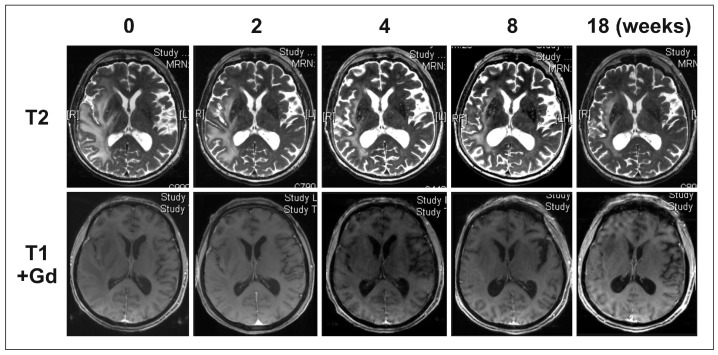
Exemplary magnetic resonance imaging (MRI) of Patient 7: non-contrast enhancing lesion. T2 sequences and T1 sequences after intravenous gadolinium administration were obtained at Weeks 0, 2, 4, 8 and 18 after BEV therapy initiation. While throughout the observation period no contrast enhancement was observed on this level, the T2 sequences from Week 18 show non-enhancing progressive disease.
